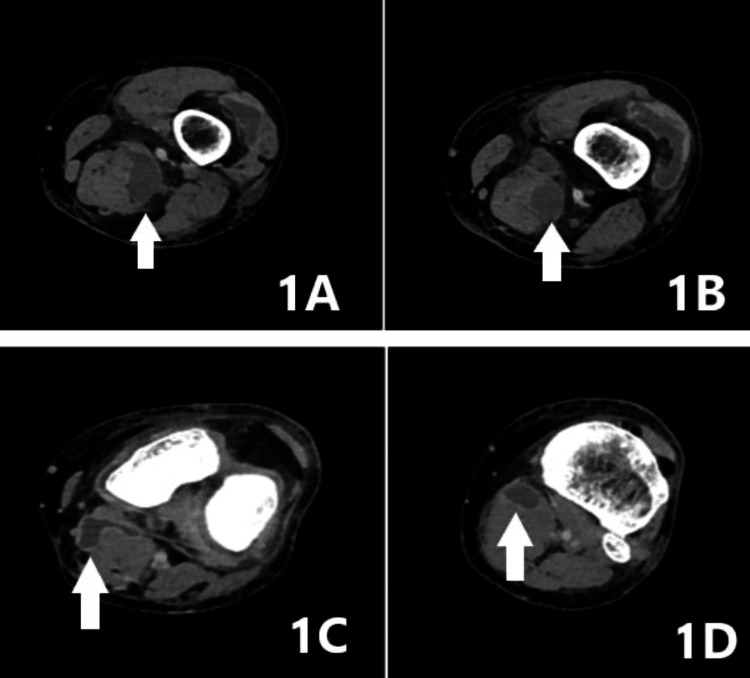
Preoperative CT scan. A. Cyst in the semimembranosus muscle (white arrow). B. Second cyst in the semimembranosus muscle (white arrow). C. Baker’s cyst (white arrow). D. Cyst in the medial head of the proximal gastrocnemius muscle (white arrow).
